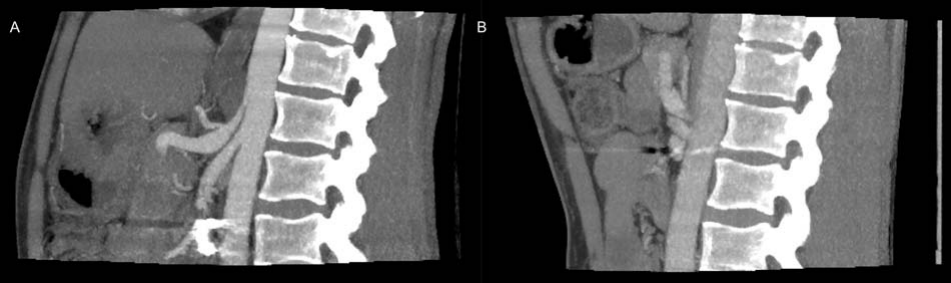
Preoperative inspiratory (A) and expiratory (B) computed tomography scan, visualizing hook formation and stenosis of the celiac artery.
